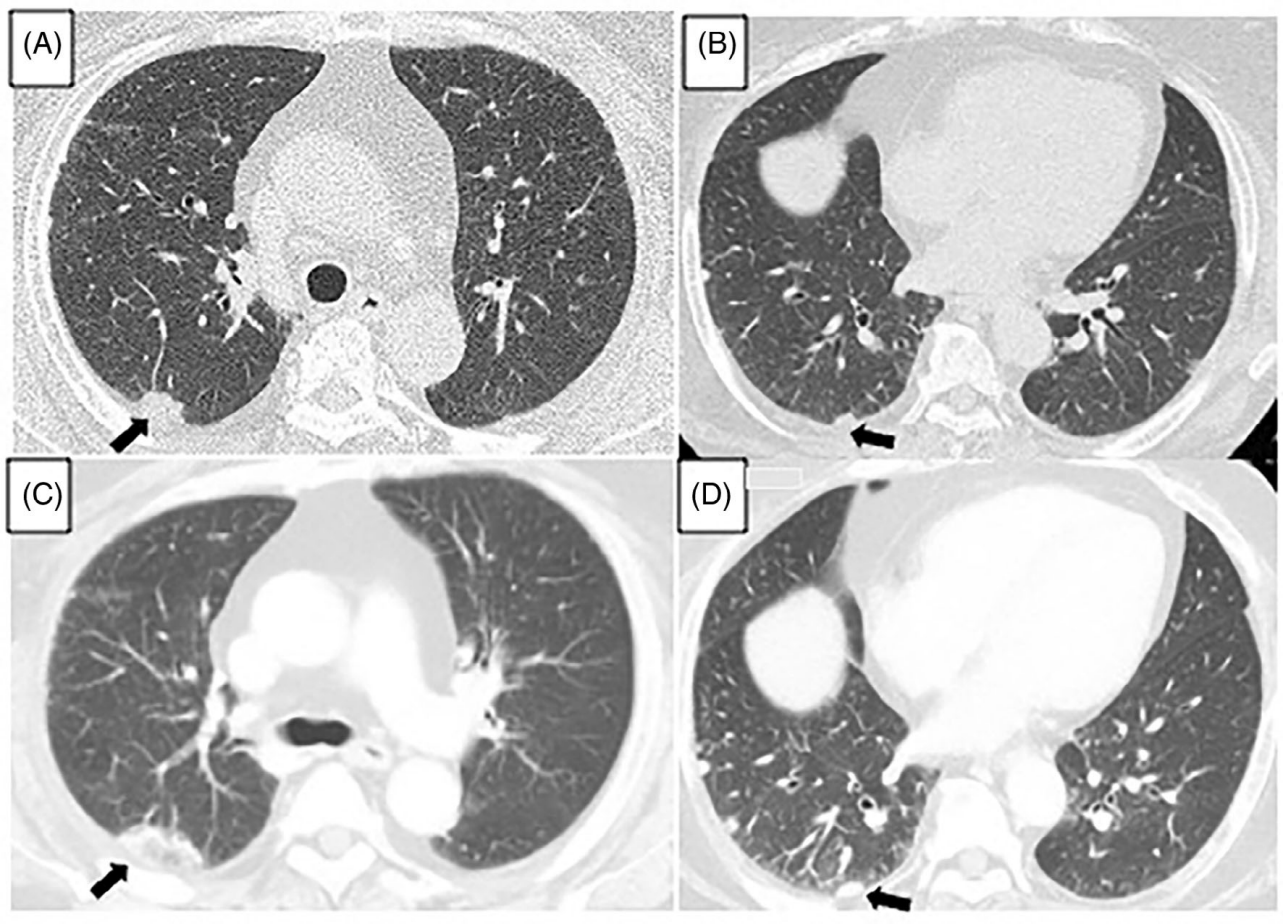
Chest CT scans showing an increase in the size and number of subpleural pulmonary nodule (A, B: at first visit; C, D: in the subsequent visit after 1 month). Arrows indicate subpleural pulmonary nodule
